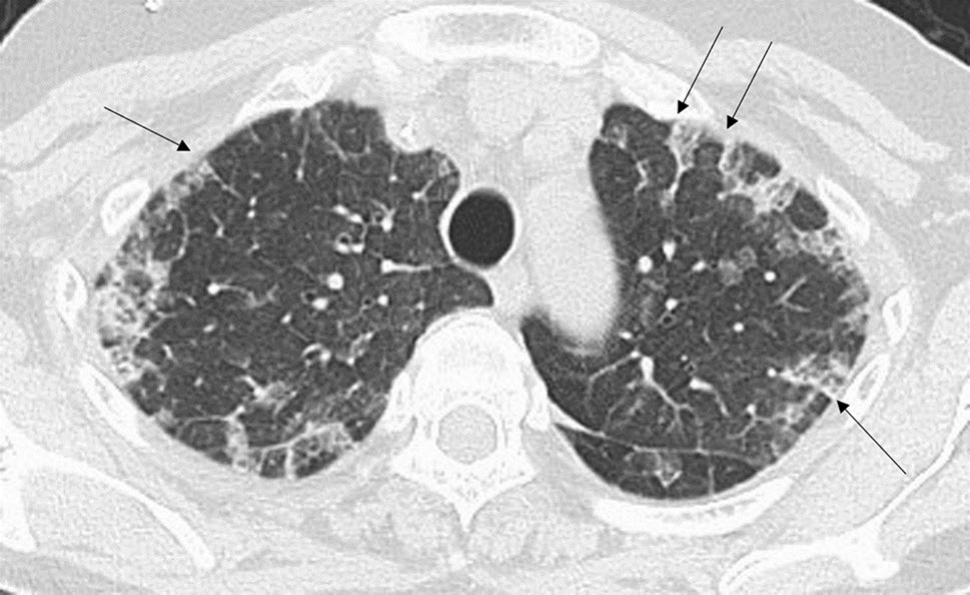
A 61-year-old woman with drug-induced pneumonia under chemotherapy for colon cancer. High-resolution computed tomography shows ground-glass attenuation with outer distribution and thickening of the interlobular septum (arrows)
